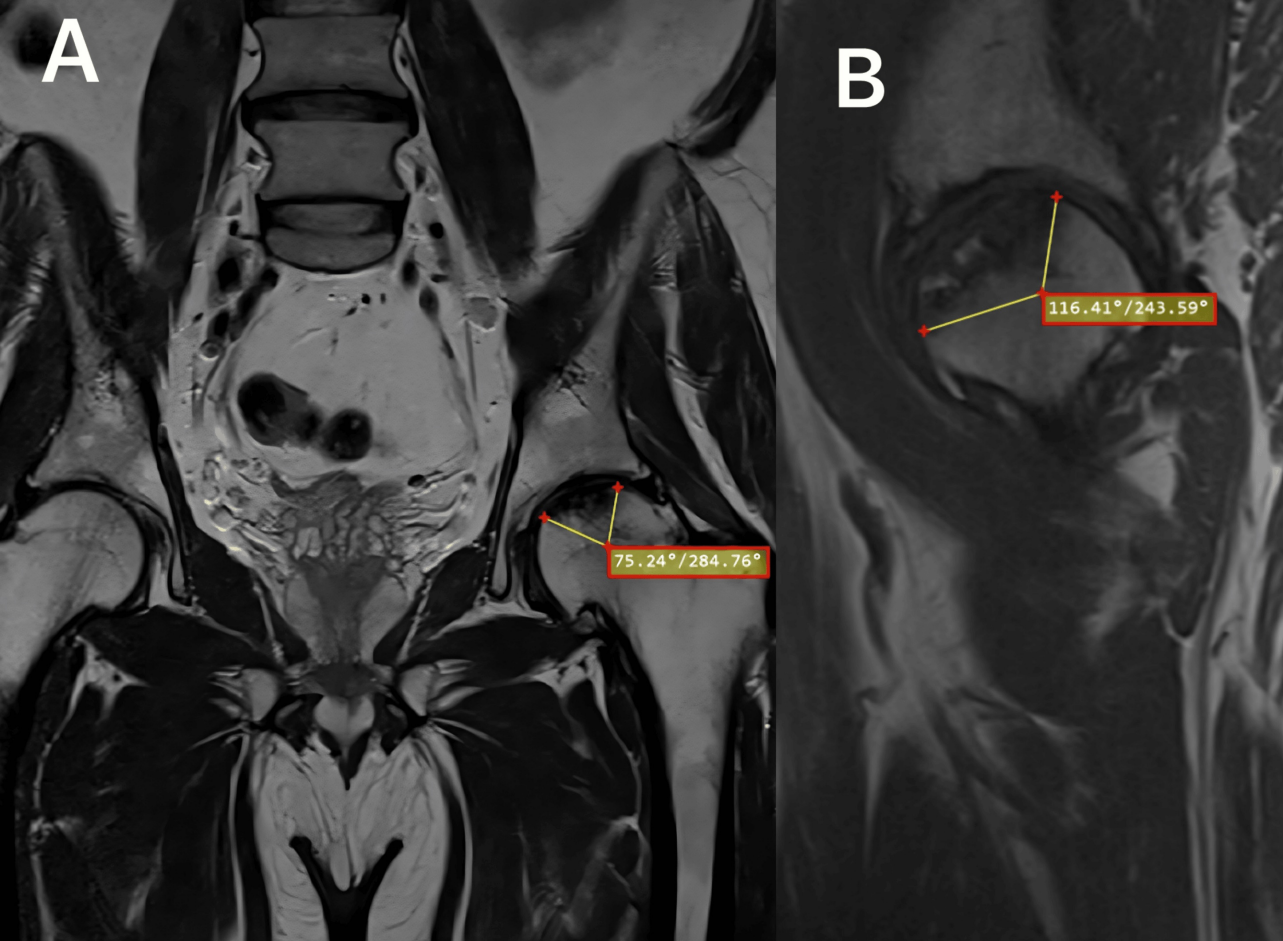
An example of the Koo and Kim method, which estimates lesion size using angles (yellow lines) measured on (A) mid-coronal and (B) mid-sagittal MRI images
